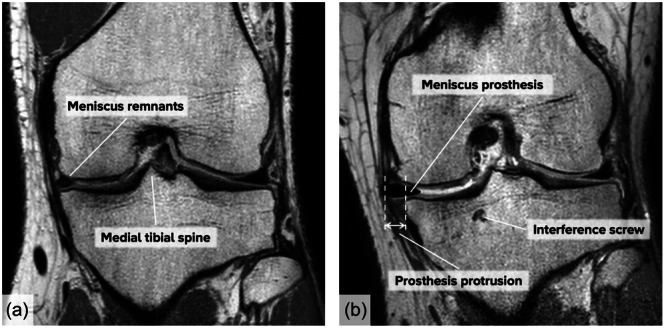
Typical example of (a) preoperative and (b) postoperative coronal MRI slices (patient 0103). The postoperative slice corresponding to the apex of the medial tibial spine was used to determine prosthesis protrusion [4]. MRI, magnetic resonance imaging.
